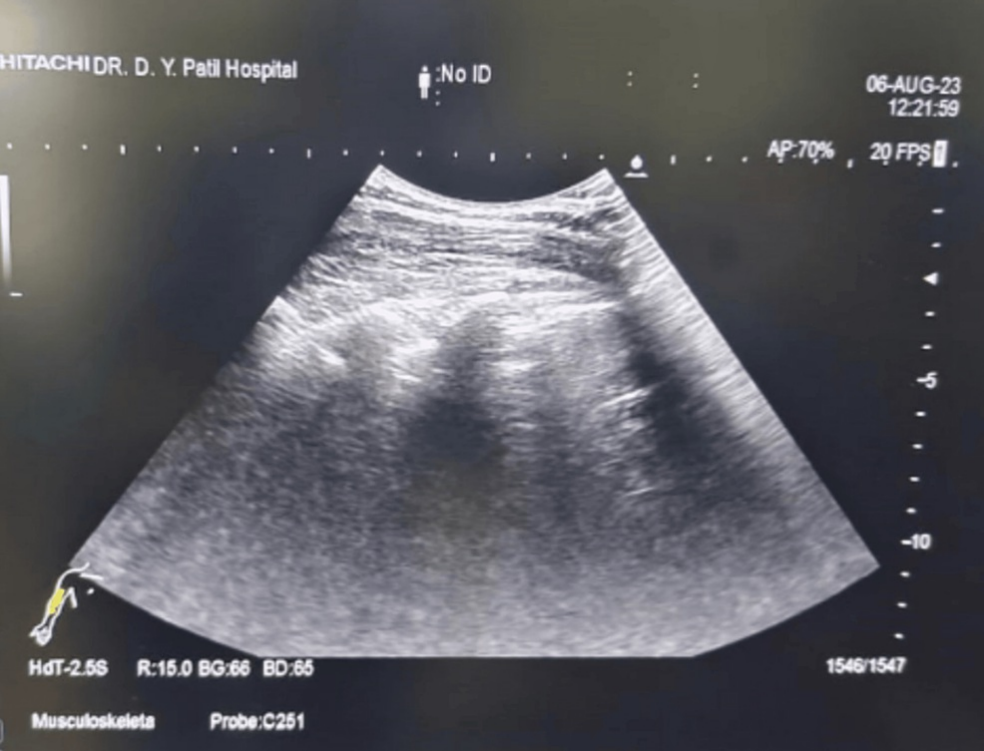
Ultrasonographic image of patient's spine in coronal and sagittal plane using a curved array probe
